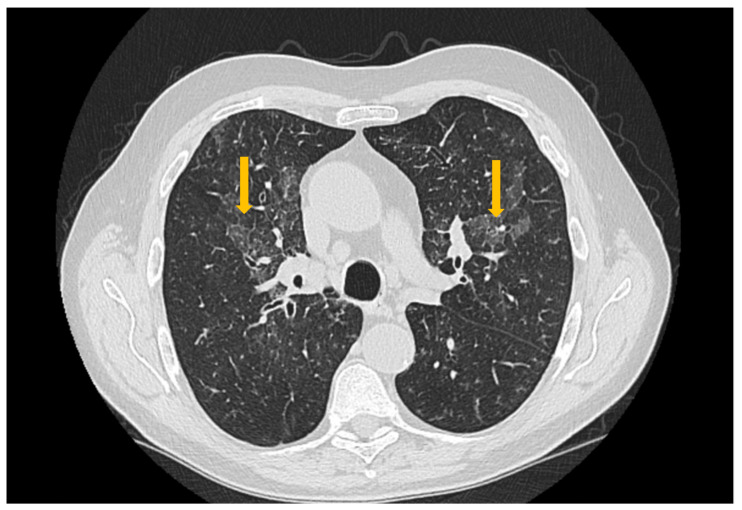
High-resolution computed tomography (HRCT) of the chest in non-specific interstitial pneumonia (NSIP). Axial HRCT image demonstrates bilateral, diffuse ground-glass opacities (arrows), associated with interlobular septal thickening and traction bronchiectasis, findings characteristic of fibrotic NSIP.
